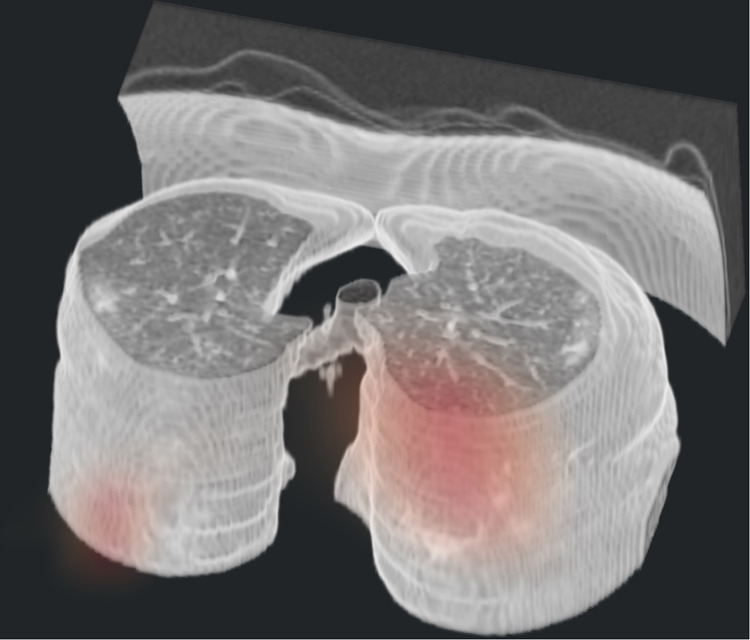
3D view of a model-generated 3D Grad-CAM superimposed on the CT of a COVID+ case with bilateral peripheral ground glass opacities and consolidation. The map was generated from only 1 forward and 1 backward pass of 1 example in the test set.
